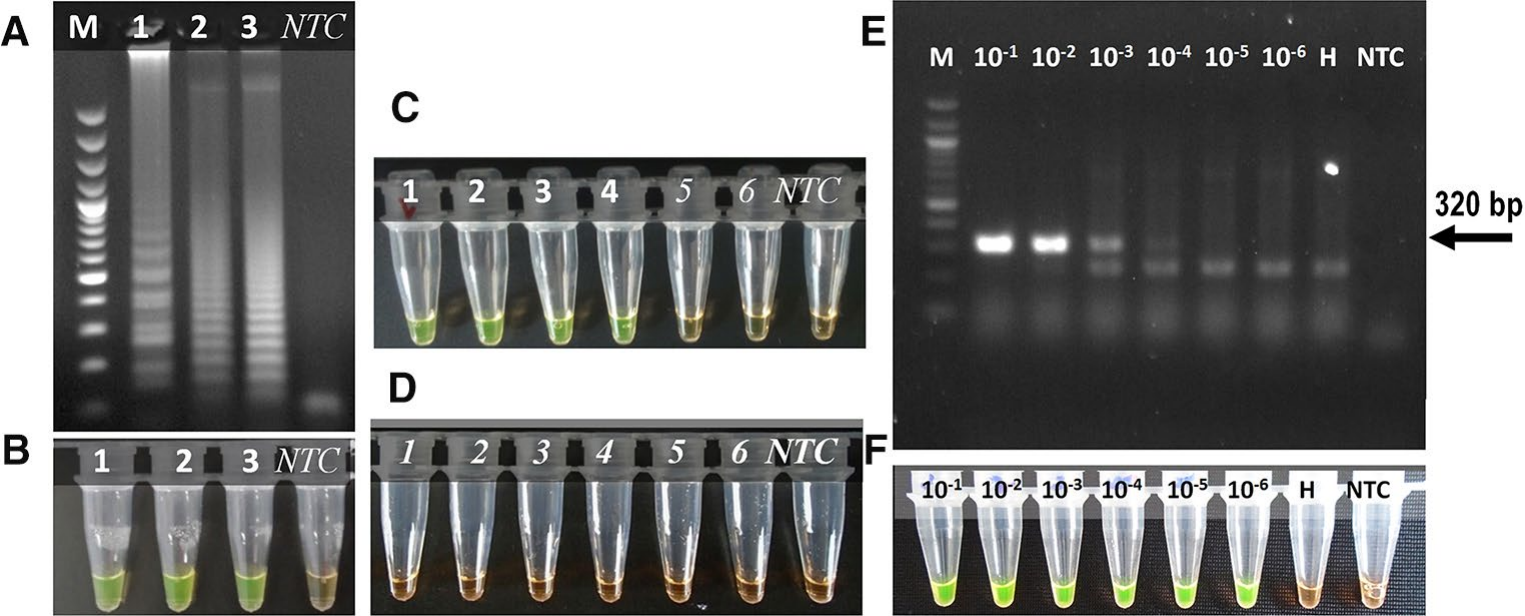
CT-RT-LAMP assays for YMV detection in yam. Amplification products were visualised by agarose gel electrophoresis (A) and with SYBR Green (B). Samples in lanes 1, 2 and 3 are YMV-infected samples. B, non-template control; M, 100-bp DNA ladder. (C) CT-RT-LAMP with loop primers. (D) CT-RT-LAMP, without loop primers. Samples in lanes 1 to 4: YMV-infected samples, 5 – 6, healthy yam samples. (E and F) Comparison between RT-PCR (E) and CT-RT-LAMP (F) for detecting YMV in infected yam leaf sample. Tenfold dilutions of total RNA from 100 ng/µl were tested. RT-PCR products were analyzed by agarose gel electrophoresis, and visual detection of RT-LAMP was done using SYBR Green dye. M, 100-bp DNA ladder; H, healthy yam RNA sample; NTC, no-template control
